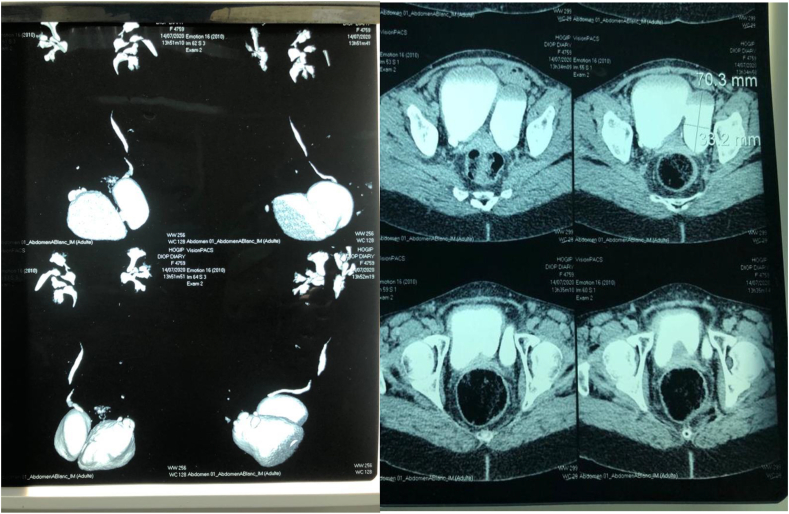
Computed tomography (CT) scan of the abdomen and pelvis showed a distended. Bladder with a thickened and irregular wall measuring up to 1 cm with the presence of a 70.3 × 33.2 mm diverticulum located at the level of the dome.
